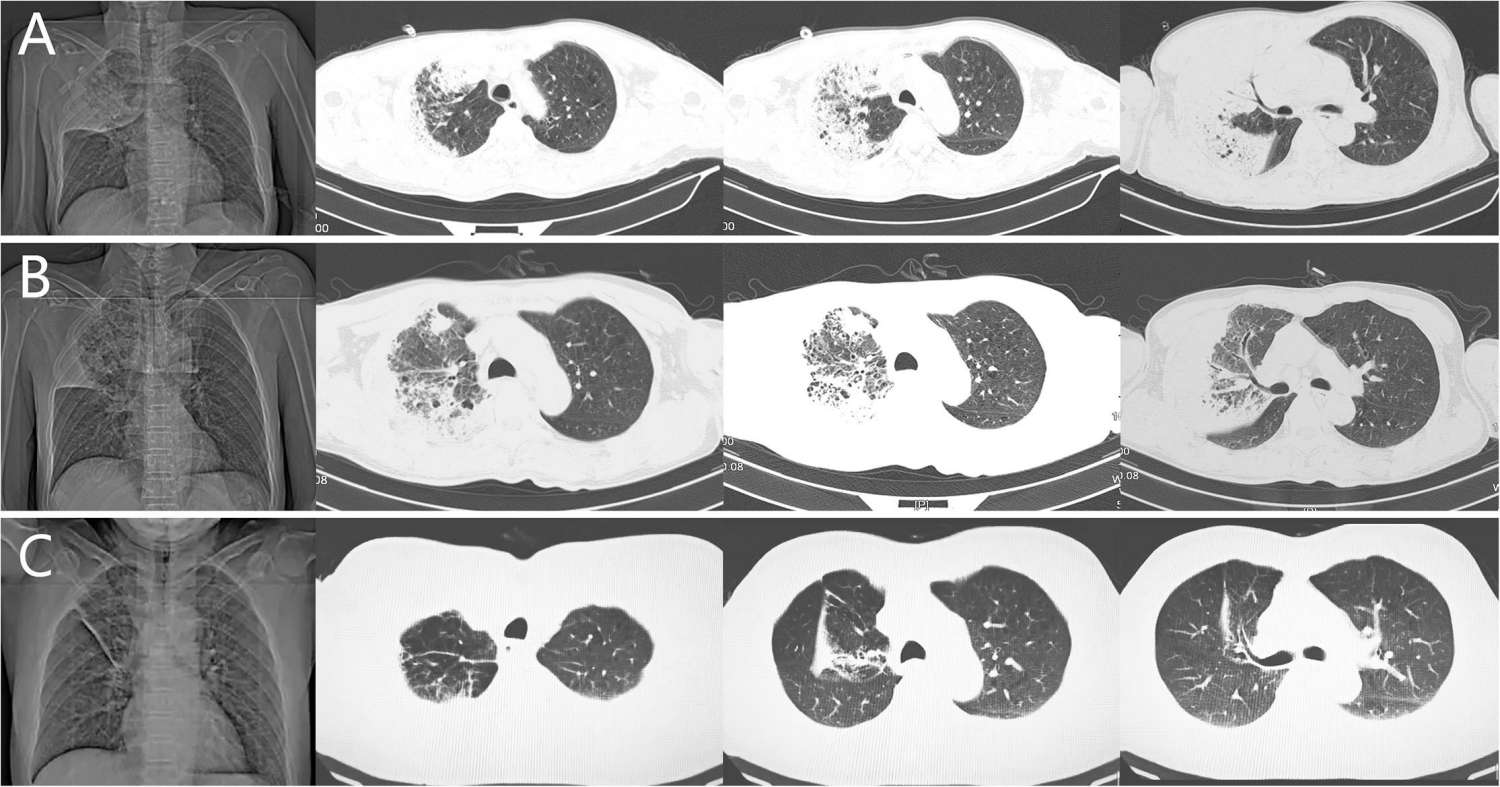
Serial roentgenograms and chest CT images of the patient we reported. (A) Transverse thin-section CT of right upper lobe showed ground-glass opacity (GGO), intralobular reticular opacity and interlobular septal thickening. Consolidation and the air bronchogram signs were also presented in the right middle lobe of the patient (on admission). (B) Chest CT of the right upper lobe revealed consolidation with cavitation and GGO, with the air bronchogram signs partially absorbed (on day 12 after admission). (C) Follow-up CT images on July 4, 2021. The consolidation with cavitation of the right upper lobe were nearly absorbed, but fiber strands were left in the right upper and middle lobe.
